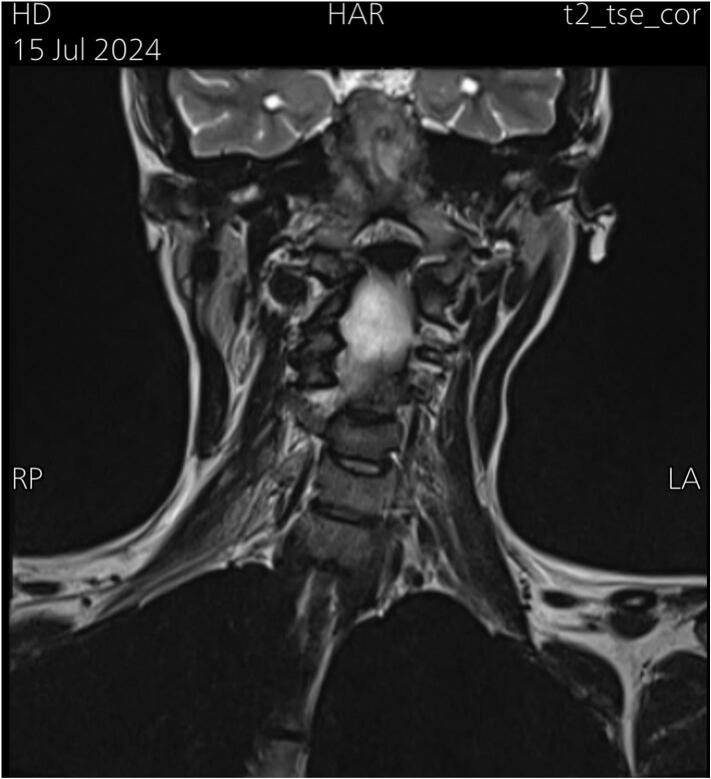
Coronal T2-weighted MRI scan of the neck showing moderate to severe rightward deviation of the lower cervical and visualized upper thoracic spine.
